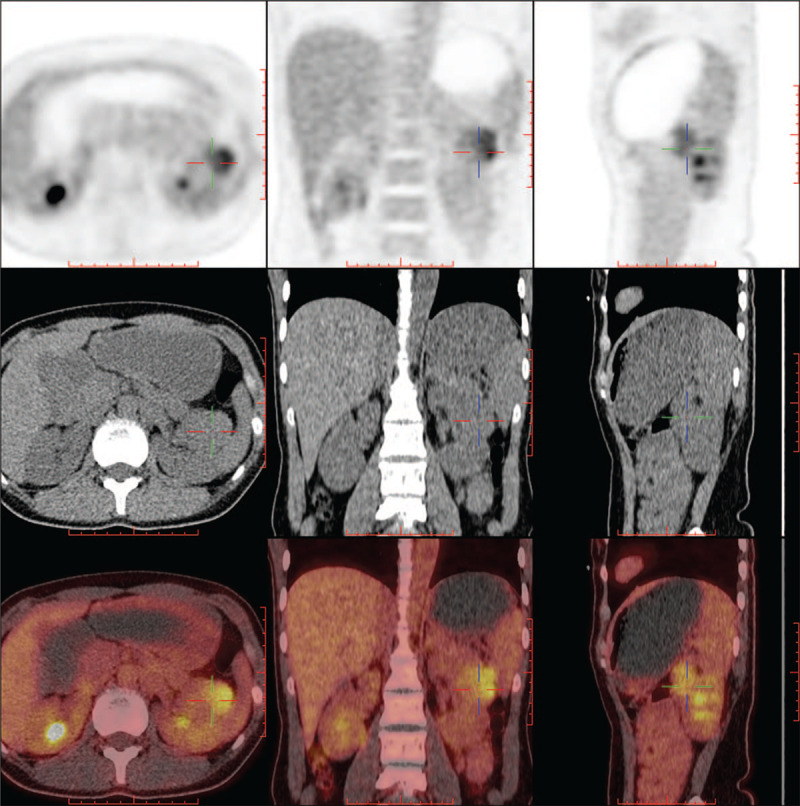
18F-FDG-PET/CT. Radioactive uptake of the tumor was abnormally increased, with a maximum standardized uptake value (SUV) of 4.6.
